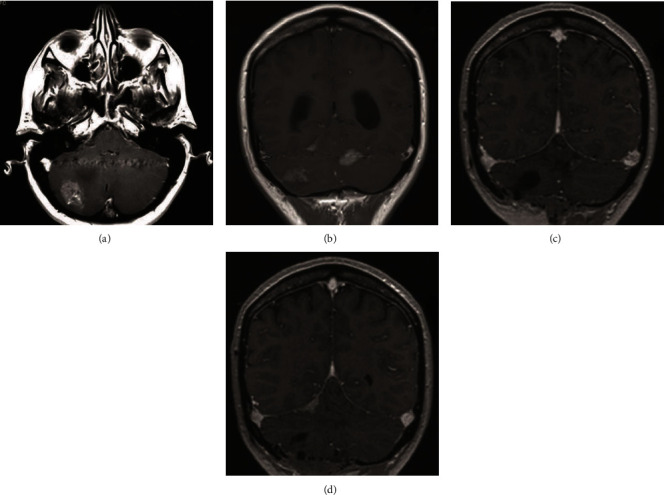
RMI T1-weighted with gadolinium. Preoperative exam shows lesions with perilesional edema, some cystic aspect and heterogeneous contrast enhancement (a, b). Postoperative exam shows as the two major lesions were excised and the small enhancing subtentorial paravermian right spot was evident (c, d).
